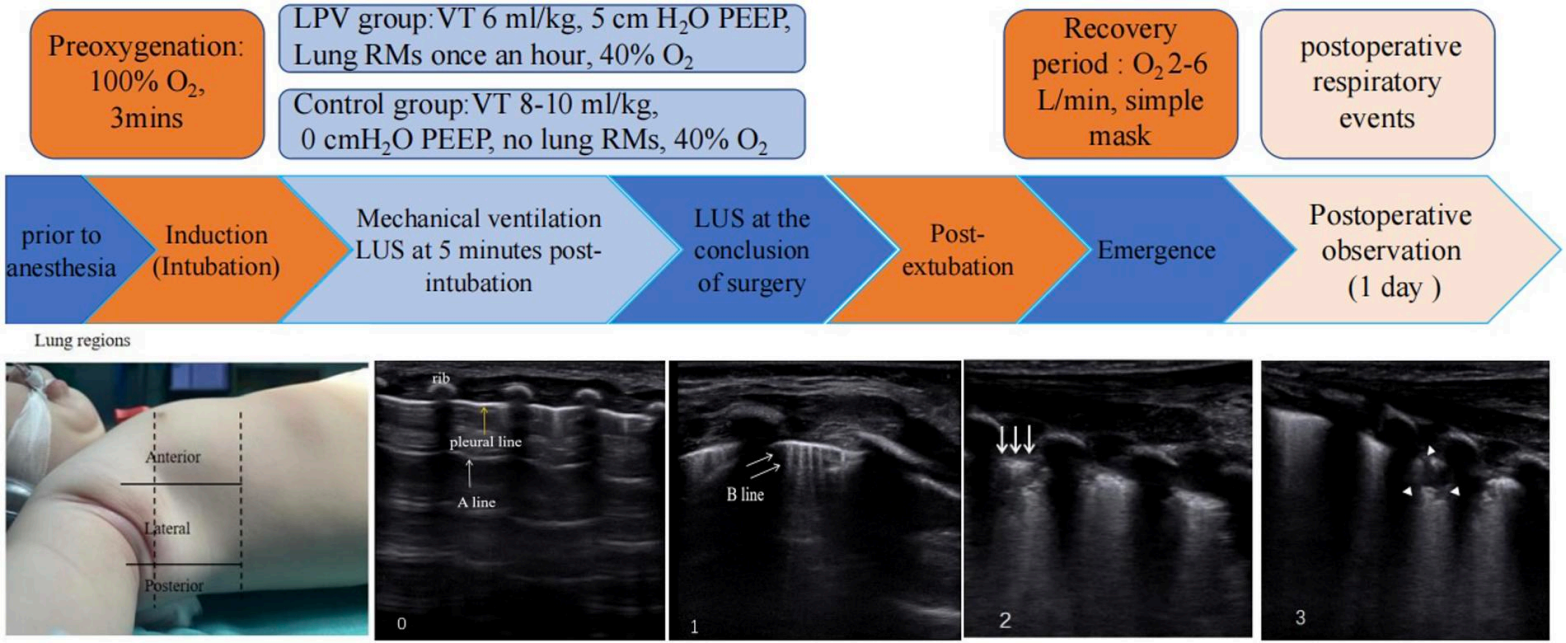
The study protocol and ultrasound lung examination region are shown. From left to right, the scores are as follows: 0, 0–2 B lines; 1, at least three B lines or one or multiple small subpleural consolidations separated by a normal pleural line; 2, multiple coalescent B lines or multiple small subpleural consolidations separated by a thickened or irregular pleural line; and 3, consolidation or small subpleural consolidation of more than 1 cm×2 cm. One yellow arrow, pleural line; one white arrow, A line; two white arrows, B line; three white arrows, subpleural consolidations; white arrowheads, consolidation.
